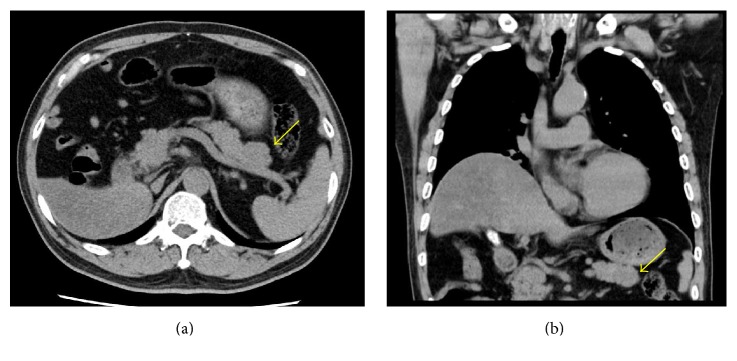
Abdominal computed tomography imaging demonstrating a 27 mm mass in the tail of the pancreas (yellow arrow).
